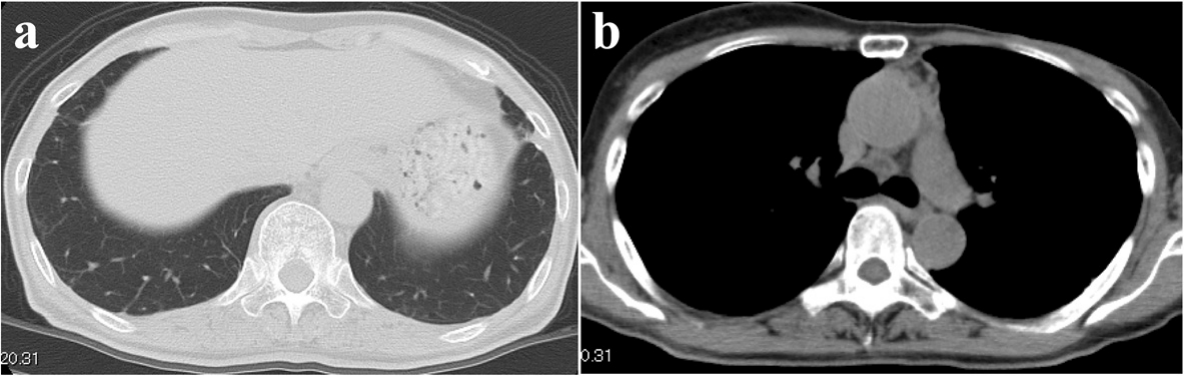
Chest computed tomography scans after trastuzumab therapy showing improvements of (a) consolidations in both the lower lobes and (b) the mediastinal mass.
